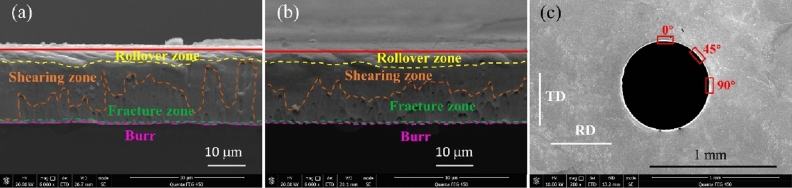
Micro-hole cross-section and its observation position. (a) Micro-hole cross-sections in non- ultrasonic punching experiments. (b) cross-sectional view of micro-hole in Micro-USF punching experiment. (c) micro-hole cross-section observation position.
